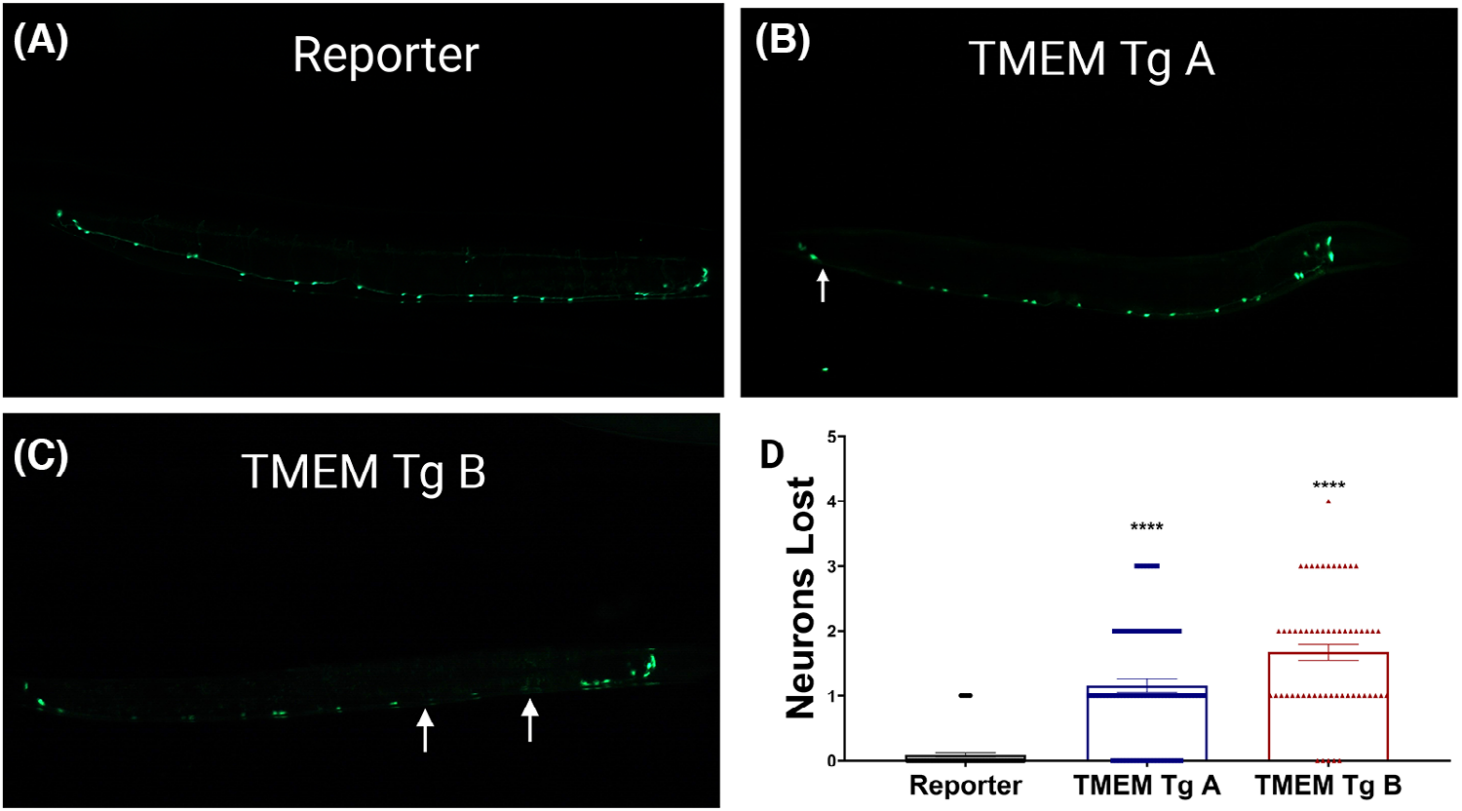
Neurotoxicity of TMEM CT in *Caenorhabditis elegans*. At day 1 of adulthood, TMEM Tg A loses on average 1 of 19 GABAergic neurons along the motor cord, while TMEM Tg B loses nearly 2 of 19 on average as visualized by the *unc‐47p*:GFP reporter (EG1285). Both Tg strains lose significantly more GABAergic neurons than the reporter strain (*p* < 0.0001). (A–C) Representative images for (A) the reporter strain, (B) TMEM Tg A, and (C) TMEM Tg B. Arrows indicate lost neurons. (D) Graphical representation of neuronal counts. *n* > 60, *N* = 3 for each strain. *p*‐Values are denoted as  for *p* < 0.0001, error bars represent standard error of the mean. TMEM CT, TMEM106B C‐terminal fragments.
